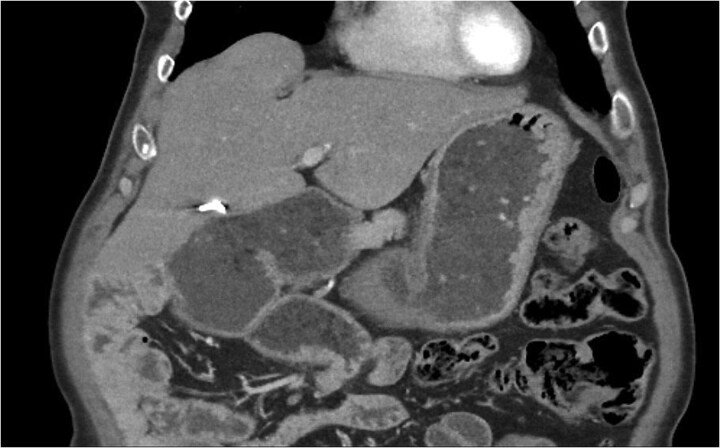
CT imaging with signs of intestinal malrotation associating important gastric and duodenal dilatation with a subsequent change in calibre at the duodenojejunal junction.
